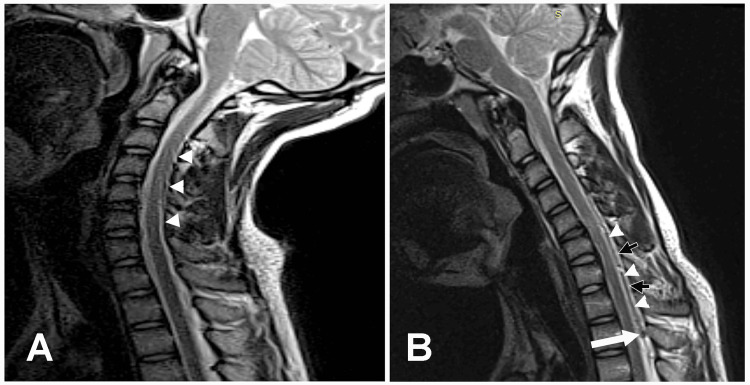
T2-weighted cervical spine MRI scan in the extended sagittal position Case 2: (A) T2-weighted cervical spine MRI scan in the extended sagittal position demonstrating the posterior dura (white arrowheads), thin epidural space from C4-C7, and no enlarged epidural veins. (B) T2-weighted cervical spine MRI scan in the flexed sagittal position revealing anterior displacement of the spinal cord and dura (white arrowheads), an enlarged epidural space from C4-C7 (black arrows), and an enlarged epidural vein (white arrow). MRI: magnetic resonance imaging
